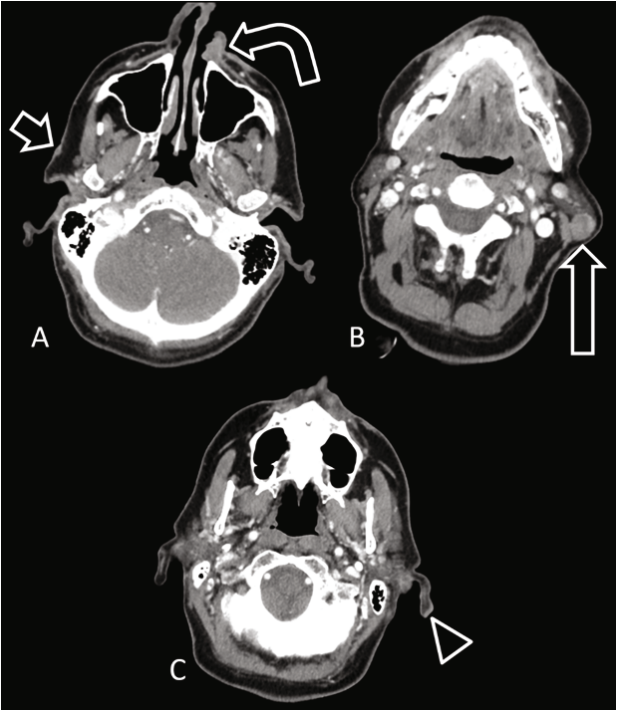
A 74‐year‐old male with a history of left nasal ala cutaneous squamous cell carcinoma (SCC), right preauricular cutaneous SCC, and left pinna melanoma presented with a palpable left upper neck mass. (A) Axial contrast‐enhanced neck soft tissue CT shows left nasal ala destruction with an enhancing soft tissue mass (curved arrow) and a small right preauricular skin lesion (short arrow). (B) An enlarged, rounded lymph node in the left parotid tail is noted. This would be an atypical site for metastatic disease from either SCC primaries. (C) Careful inspection shows subtle right inferior helix skin thickening (arrowhead), which would be easy to overlook if the history of melanoma was unknown. Parotid node fine needle aspiration followed by partial parotidectomy confirmed metastatic melanoma.
